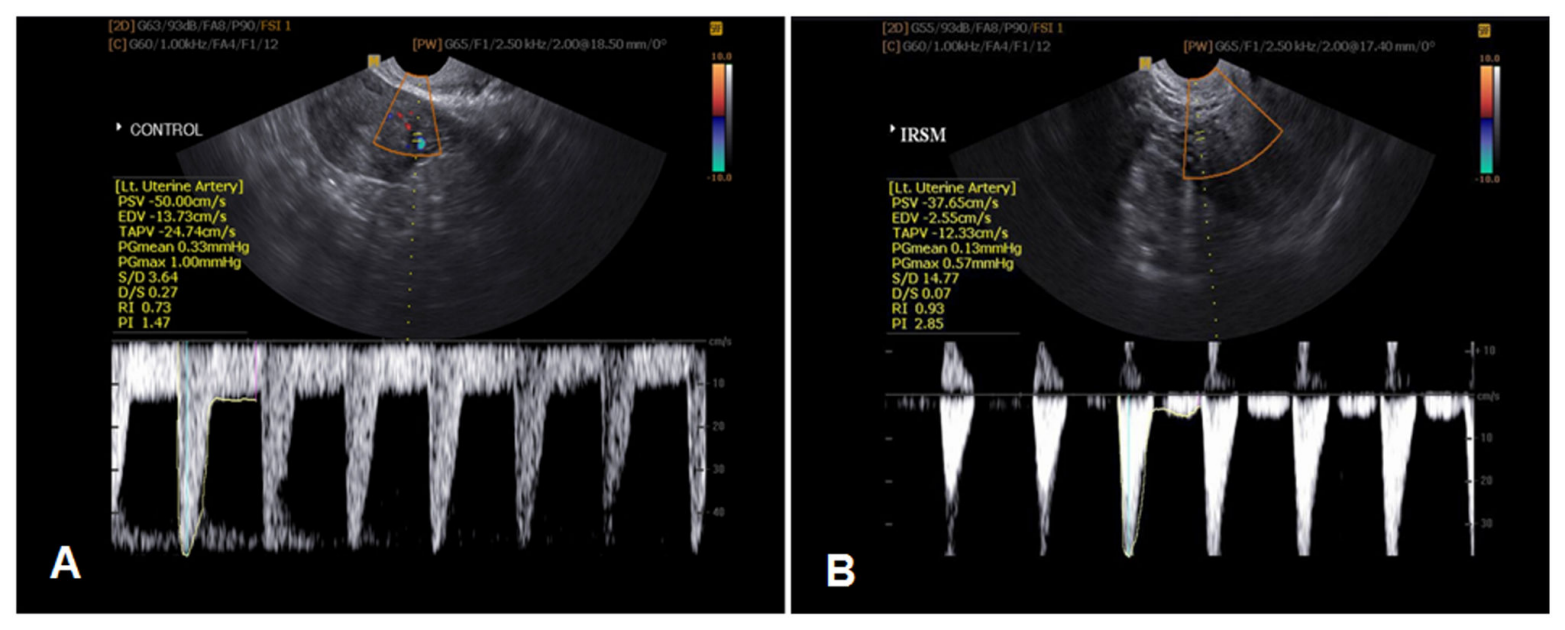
Typical 2D Doppler ultrasound image of sub-endometrial blood flow measured in (A) controls and (B) IRSM.
